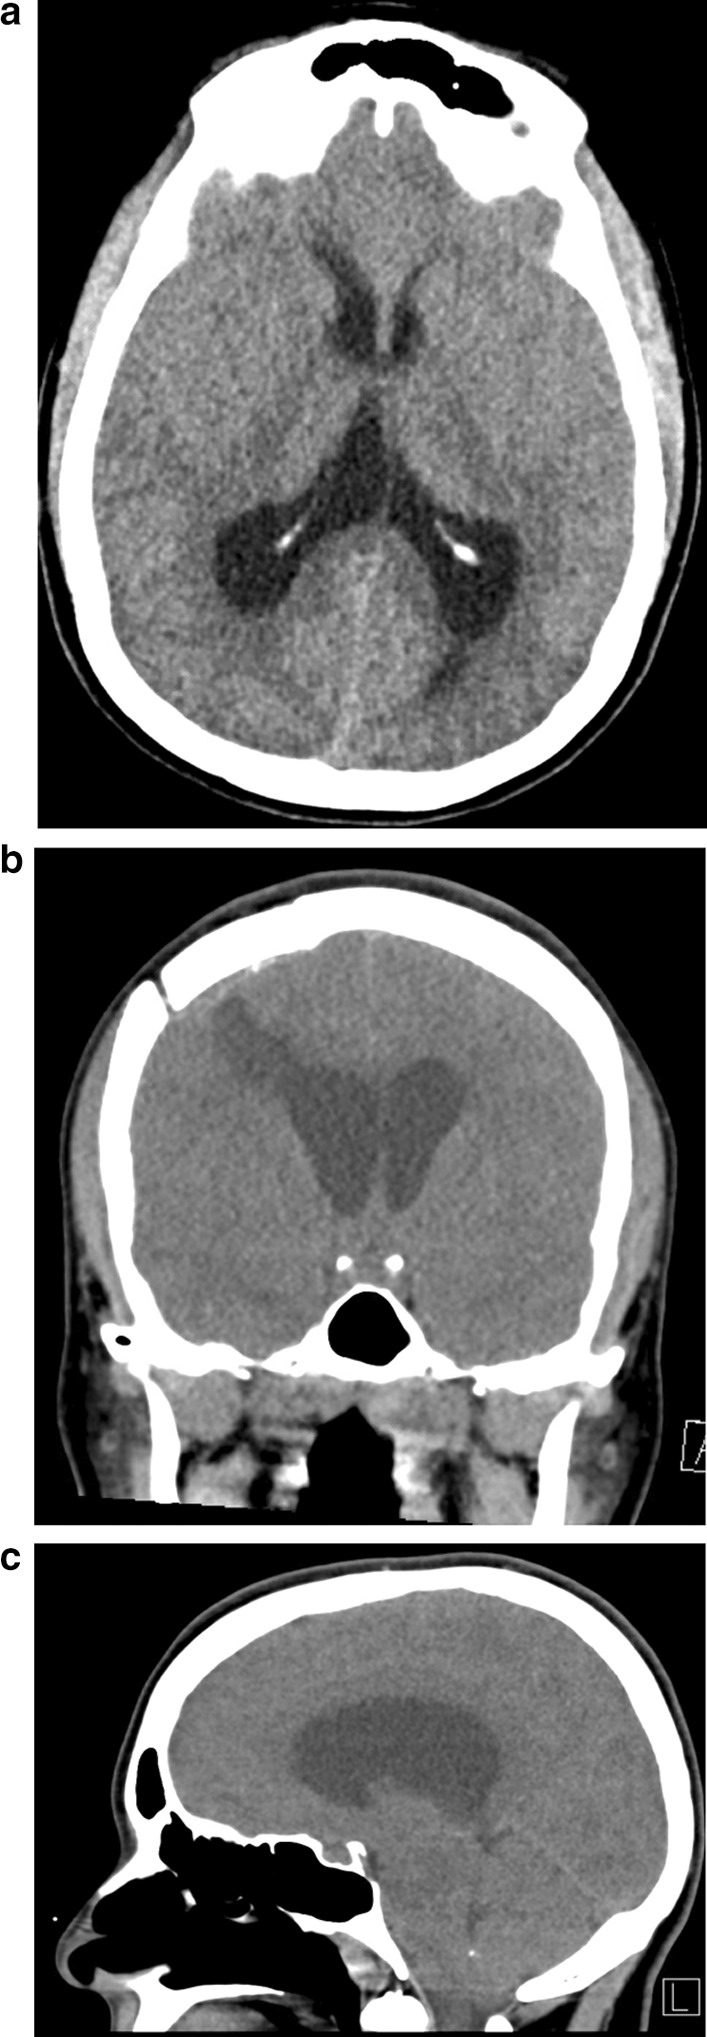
Axial (a), coronal (b), and sagittal (c) CT scan demonstrating resection of the colloid cyst and dilatation of the lateral ventricles on presentation to the ED 6 weeks postoperatively. Note that the third and fourth ventricles are not dilated, suggestive of obstructive hydrocephalus
